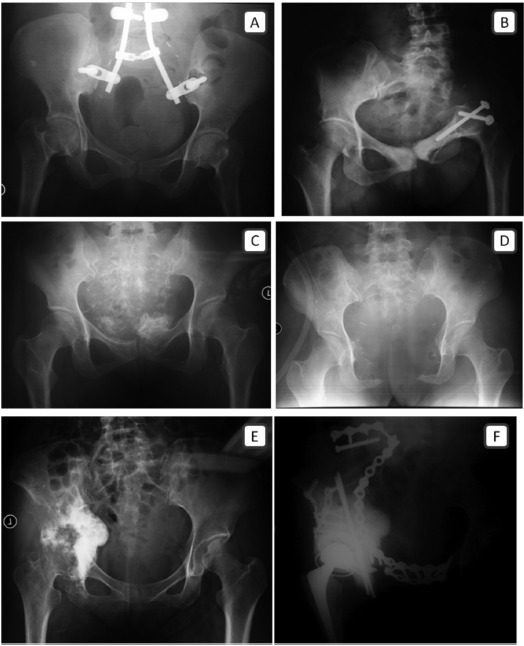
Anteroposterior plain radiographs of the pelvis. Galveston iliolumbar fusion following iliosacral resection (A). Ischiofemoral
